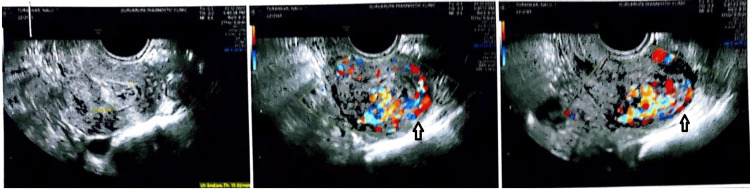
Uterine AVM on color Doppler The arrow depicts enhanced myometrial vascularity with evidence of other hyperechoic areas. AVM, arteriovenous malformation
